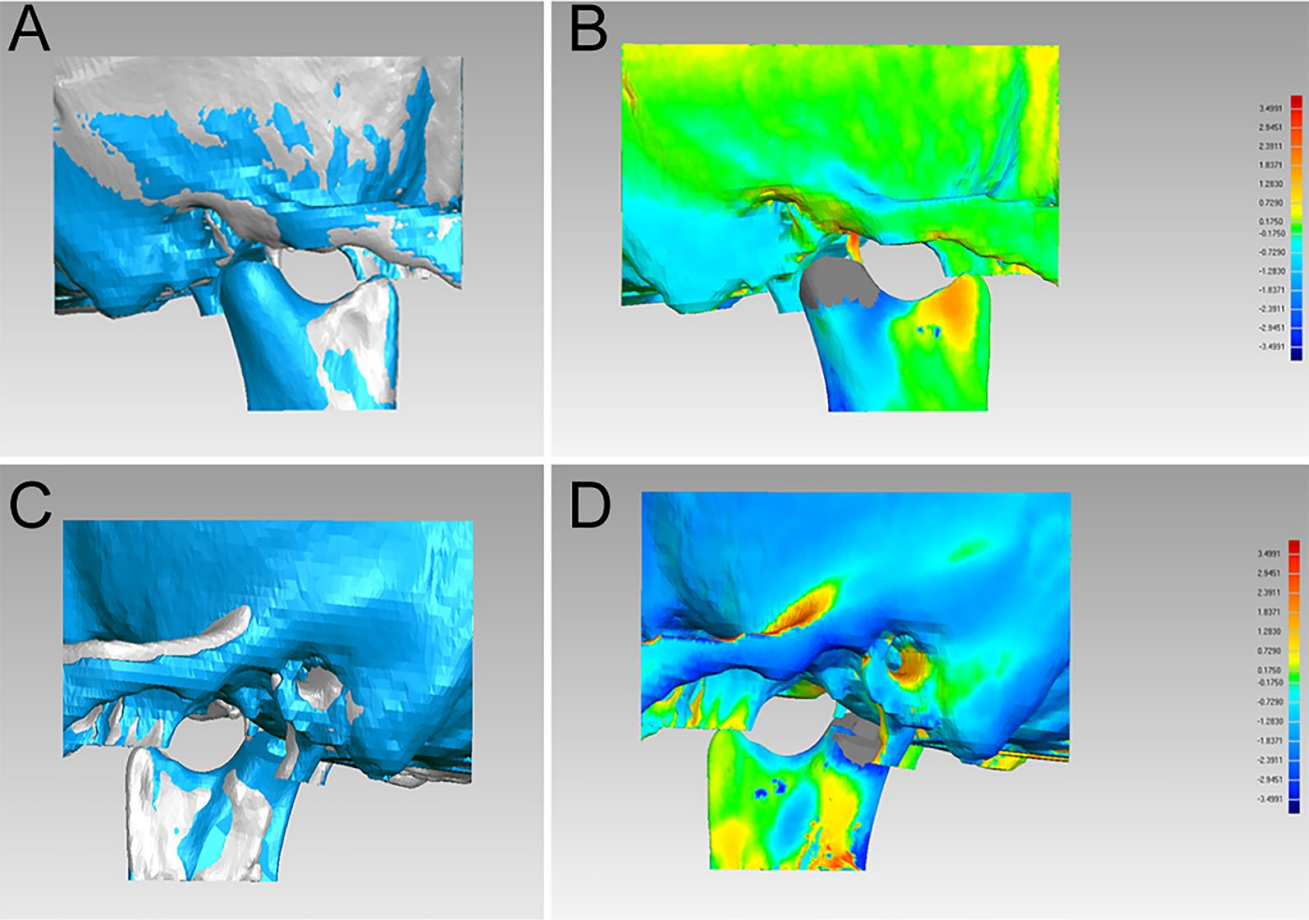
Evaluation of the stability of condylar repositioning guide. a, c superimposition of postoperative CT scan at 3 days after surgery (grey) and postoperative CT scan at 1 year after surgery (blue); b, d the postoperative stability study of condylar repositioning guide using the Geomagic Studio 2013 software. e, f the magnification view of part of zygomatic arch, fossa, and neocondyle
